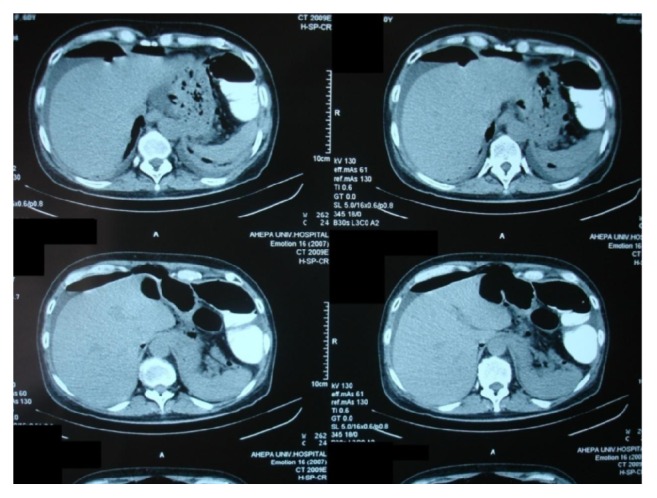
Abdominal CT scan. Perihepatic collection with free abdominal gas.
